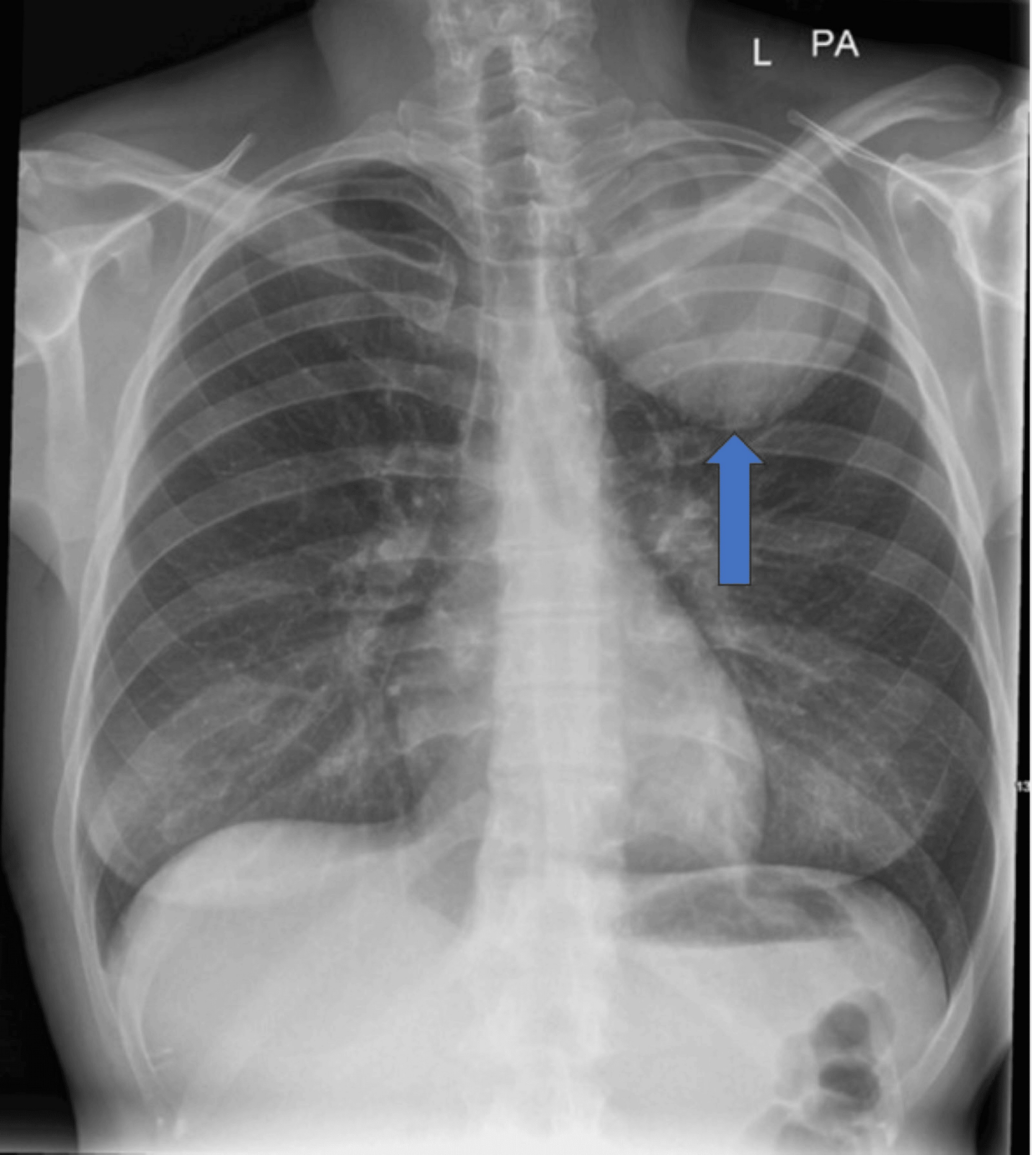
Chest X-ray showing a 10 cm rounded homogenous opacity at the left apex
